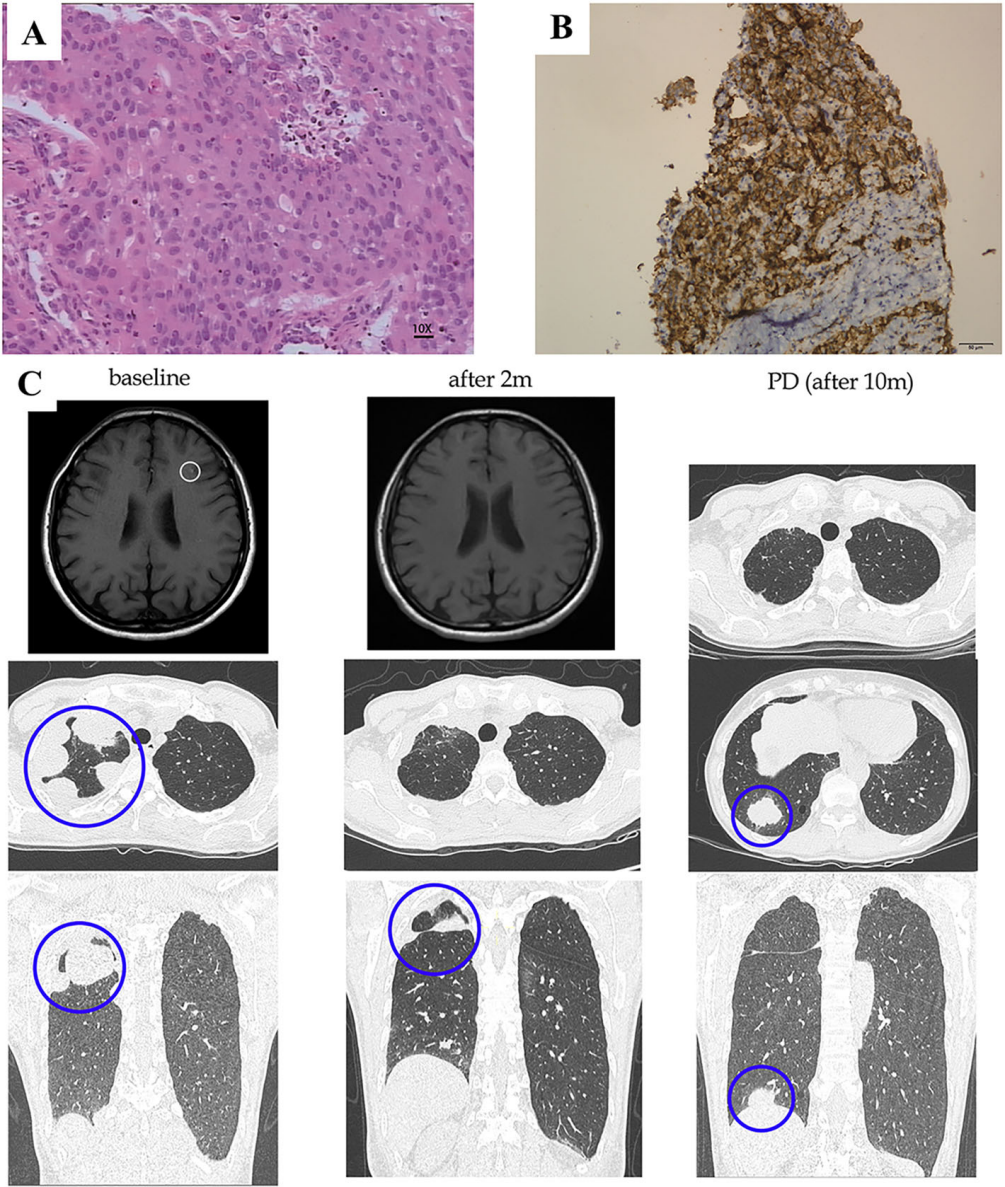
Response to pembrolizumab in a case with KIF5B-RET fusion and a high level of PD-L1 expression. a Adenocarcinoma nature (10×). b High level of PD-L1 expression (TPS = 50%). c Computed tomography scan and magnetic resonance imaging revealing the clinical response to pembrolizumab. Abbreviations: PD - progression disease
